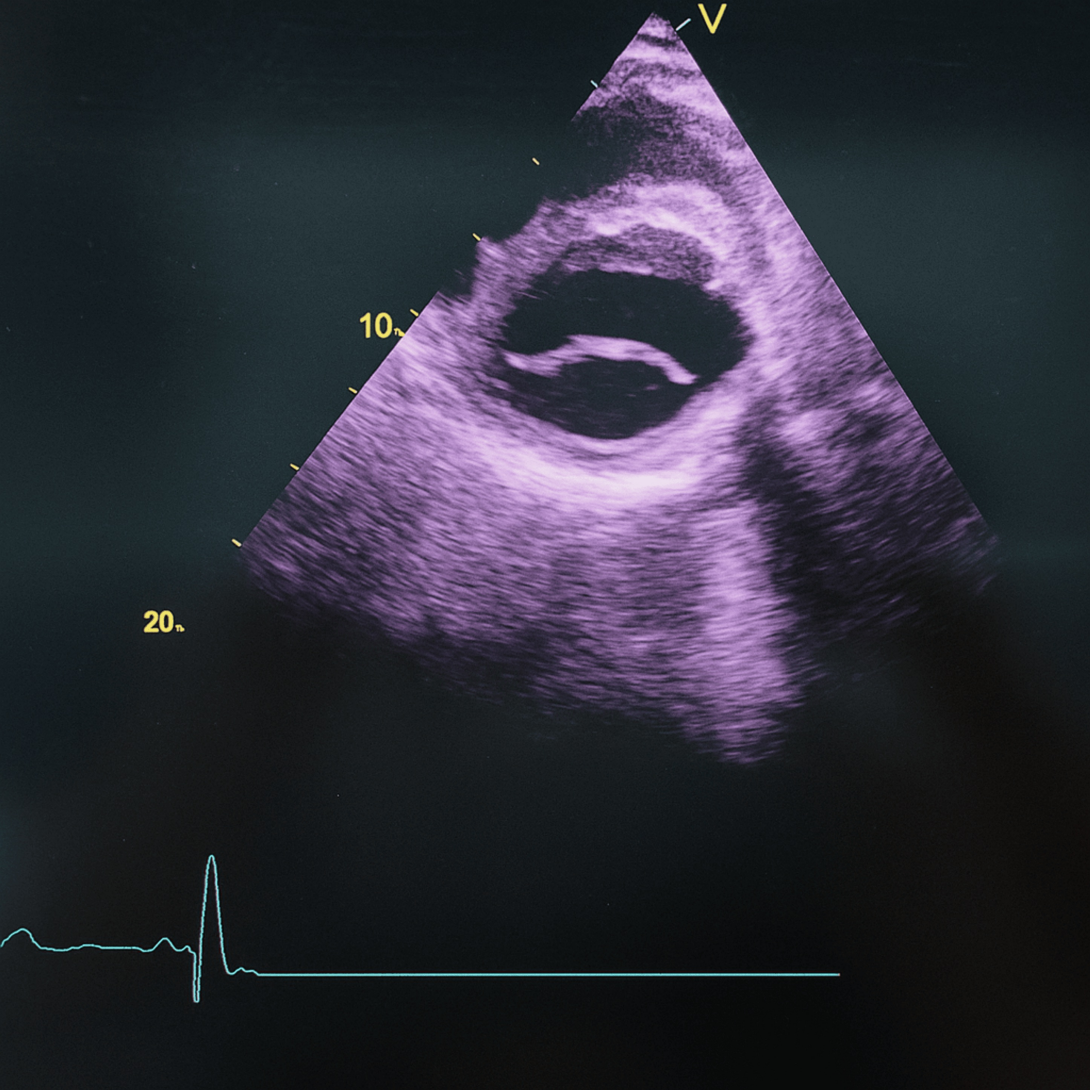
Echocardiography showing a bicuspid aortic valve
